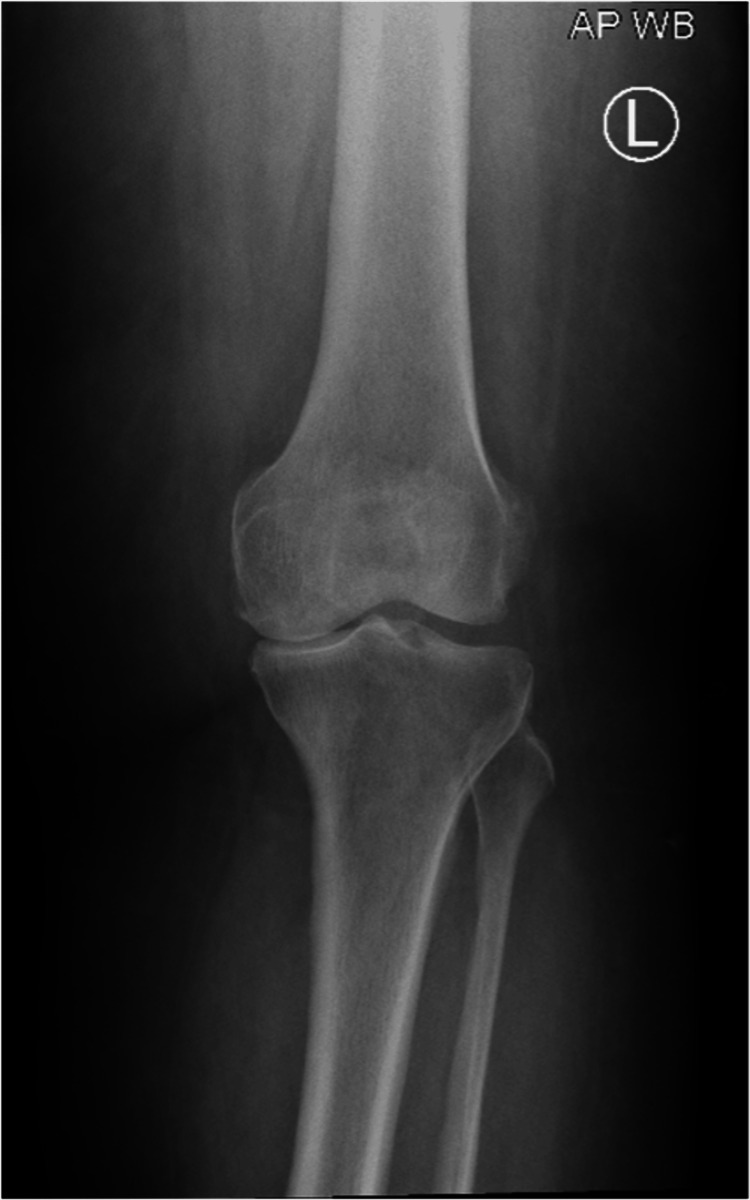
Left knee X-ray before total knee arthroplasty showing osteoarthritis
